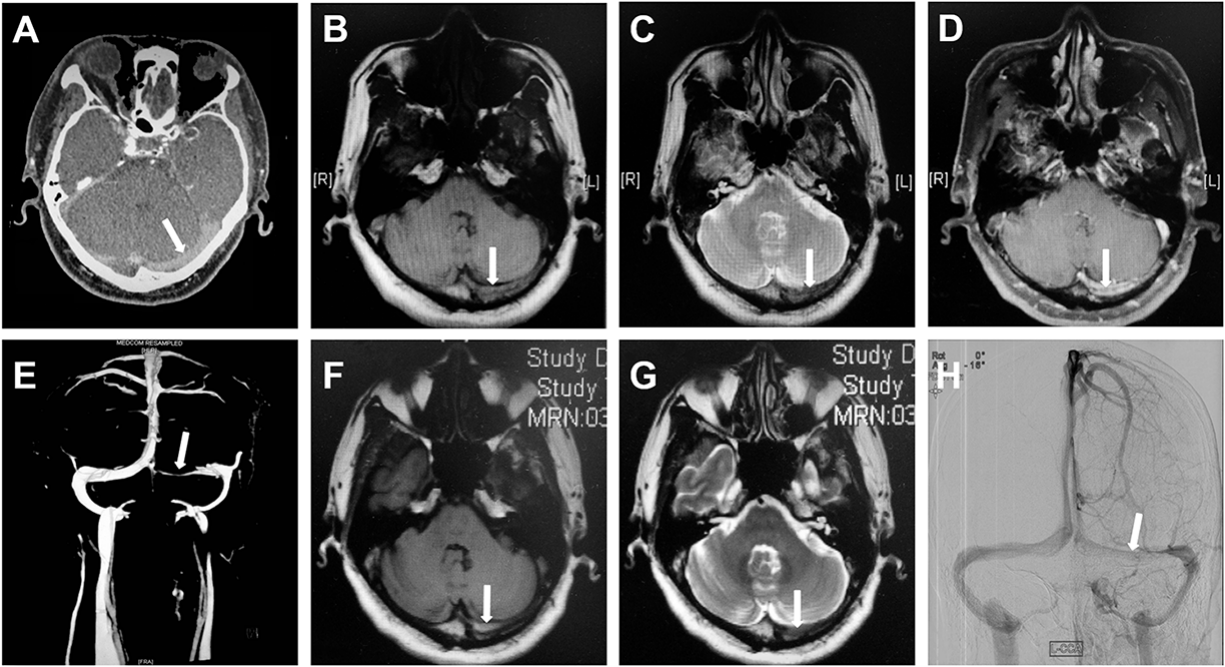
Double-track sign and the varied signal of thrombus. A 46-year old female patient with left transverse sinus stenosis. Hyperdensity of thrombus (cord sign) on non-contrast CT (A). Thrombus was isointense on T1WI (B) and hypointense on T2WI (C) which demonstrated an acute stage of thrombus. Double-track sign (arrow) was detected on axial Gd-enhanced T1WI (D). Severe stenosis was confirmed on MRV which showed a linear signal of blood flow in the left transverse sinus (E) (Time from MRI to MRV was 2.5 hours). No remission in headache in the patient after anticoagulant therapy for one week, and follow-up scan showed that the thrombus was hyperintense on T1WI (F) and hypointense on T2WI (G), which demonstrated an early sub-acute stage of thrombus. Transverse sinus stenosis was confirmed on DSA which showed the filling deficiency in the left transverse sinus (H) (Time from MRI to DSA was 3 hours).
